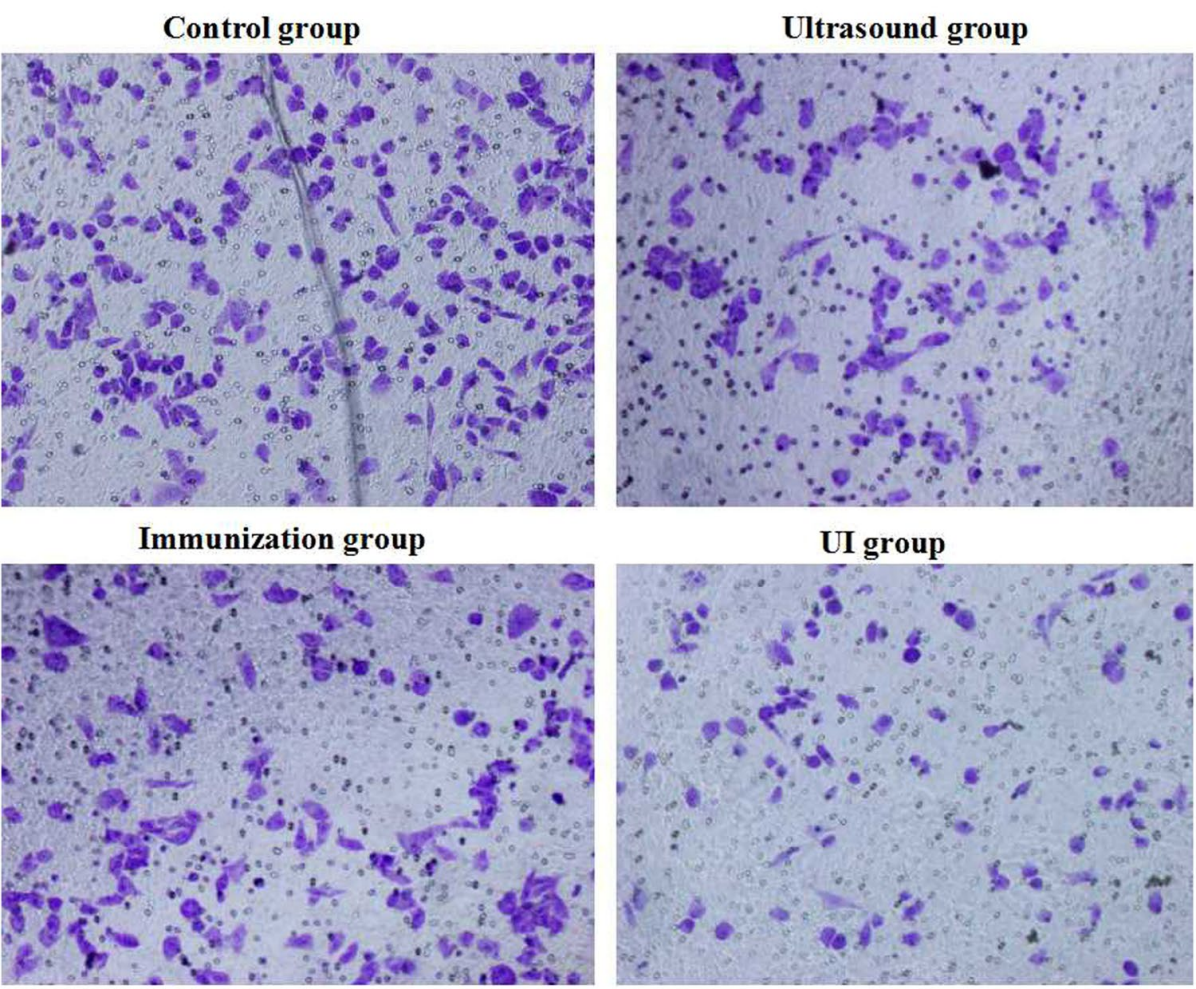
Numerous cells passed through the Matrigel matrix in the control group; fewer cells of the immunization and ultrasound groups invaded; cell invasiveness in the UI group was the weakest (×200).
